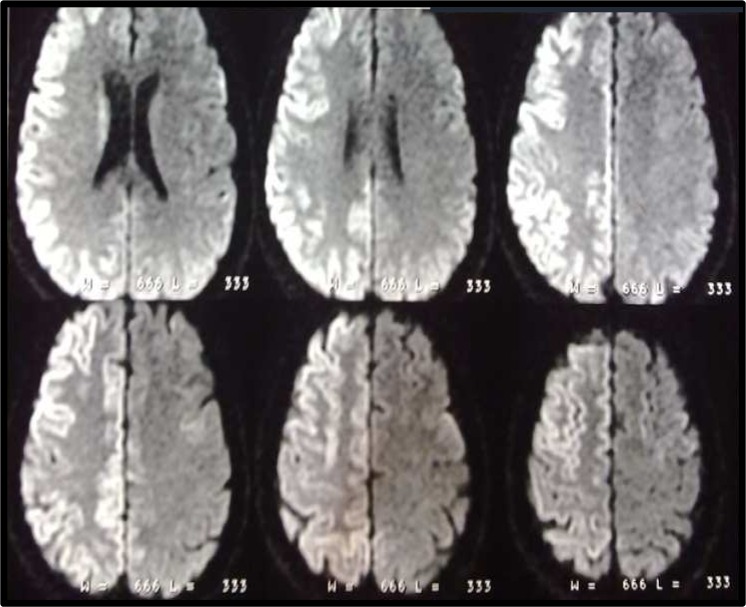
The brain magnetic resonance imaging diffusion (weighted imaging) shows right side diffuse cortical hyperintensity
